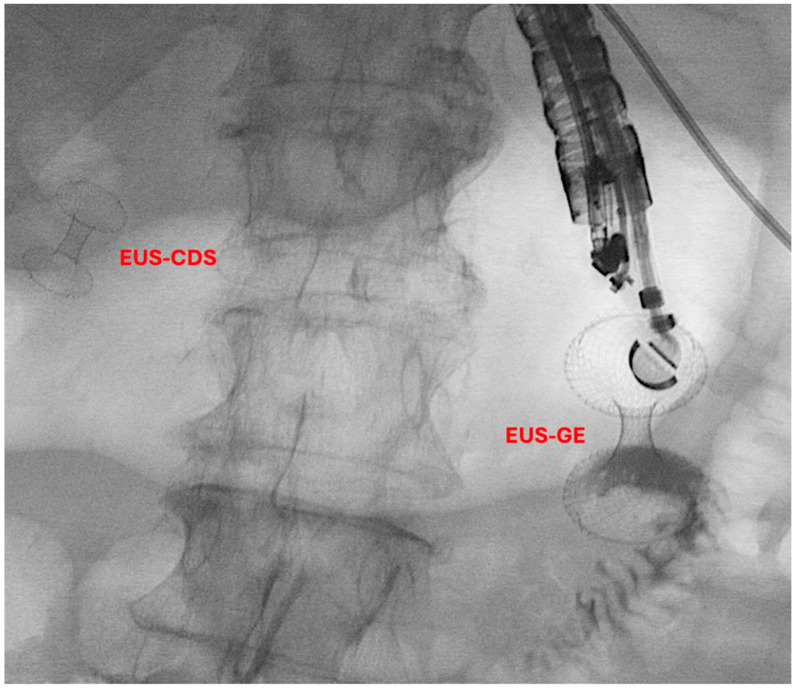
Fluoroscopic image of a patient presenting with malignant double obstruction (biliary and gastric outlet) wherein Endoscopic Ultrasound-Guided Choledochoduodenostomy (EUS-CDS) and Endoscopic Ultrasound-Guided Gastroenterostomy (EUS-GE) have been performed (permission has been taken from Dr. Jayanta Samanta for the publication of this image). The copyright of the image belongs to the authors.
